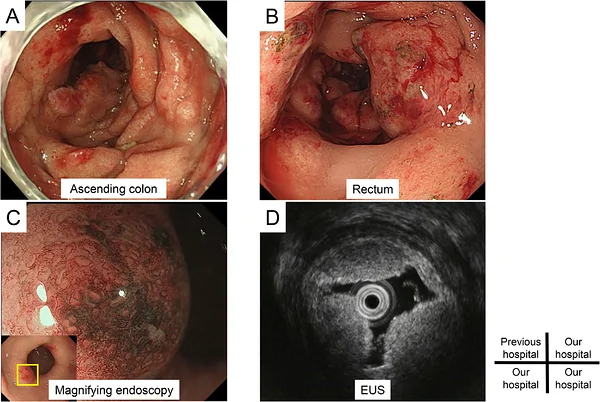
Colonoscopy and endoscopic ultrasonography (EUS) findings: (A) Colonoscopy at the patient's previous hospital revealed multiple subepithelial lesion (SEL)‐like elevations in the colon; (B and C) repeat colonoscopy at our hospital demonstrated progression of the SEL‐like elevations, whereas the mucosal surface architecture remained preserved; and (D) EUS depicted thickening centered in the second layer.
